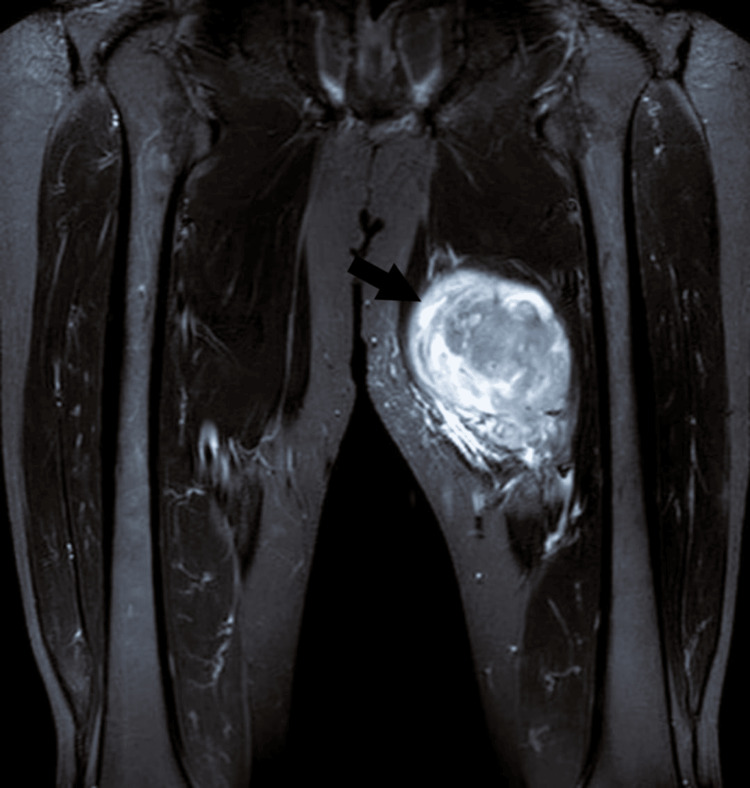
Axial T2-weighted MRI showing a hyperintense, well-defined mass in the thigh with surrounding edema.
